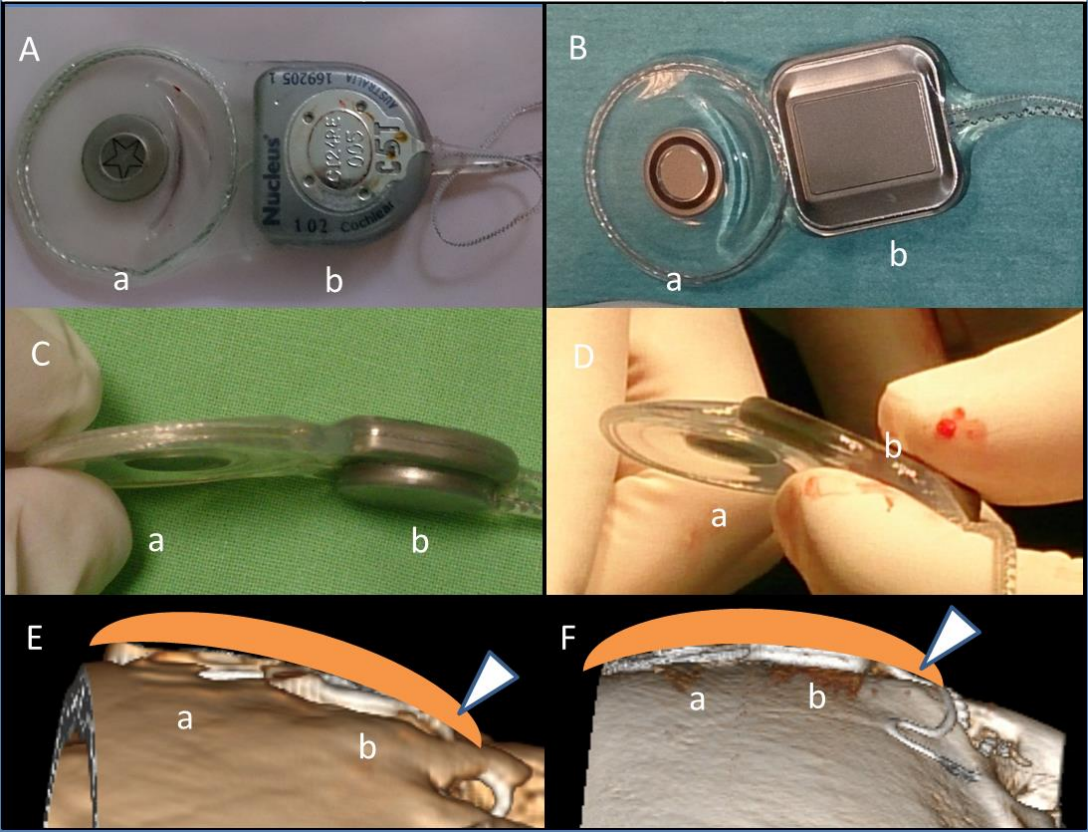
Cochlear™ Nucleus® Freedom (on the left) and Cochlear™ Nucleus® Profile (on the right) implants from above (A and B) and side view (C and D). The dimensions are detailed in the table. E and F show the three dimensional CT reconstructions (software: RadiAnt DICOM Viewer). The electronics capsule (a) and the antenna (b), in the middle of which a magnet is mounted, can be easily recognized. The arrowhead represents the soft tissue over the implant. The profile of the implant protrudes out of the bony surface only as much as the earlier type of implant.
